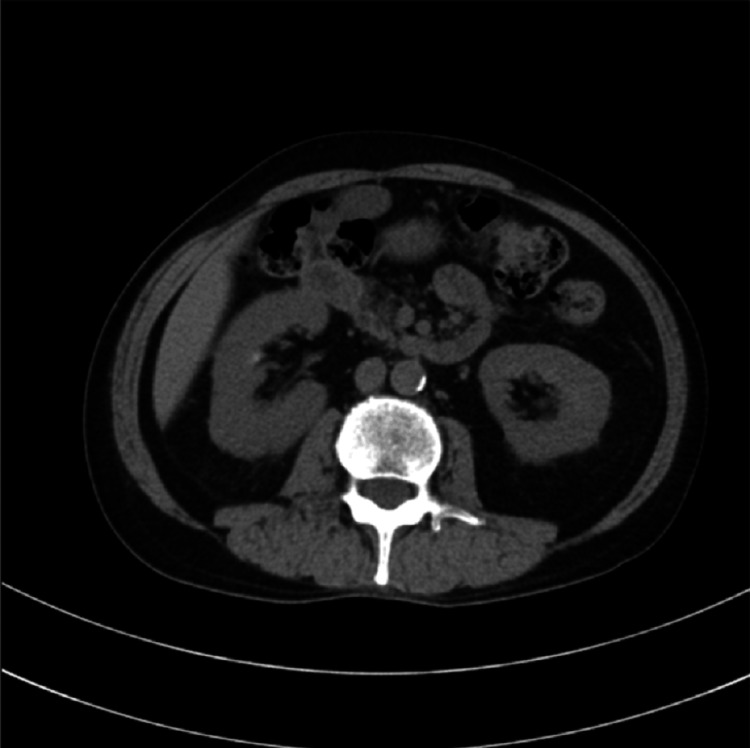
Metastatic sites in patient 2 Computed tomography imaging revealed no apparent visceral metastases.
